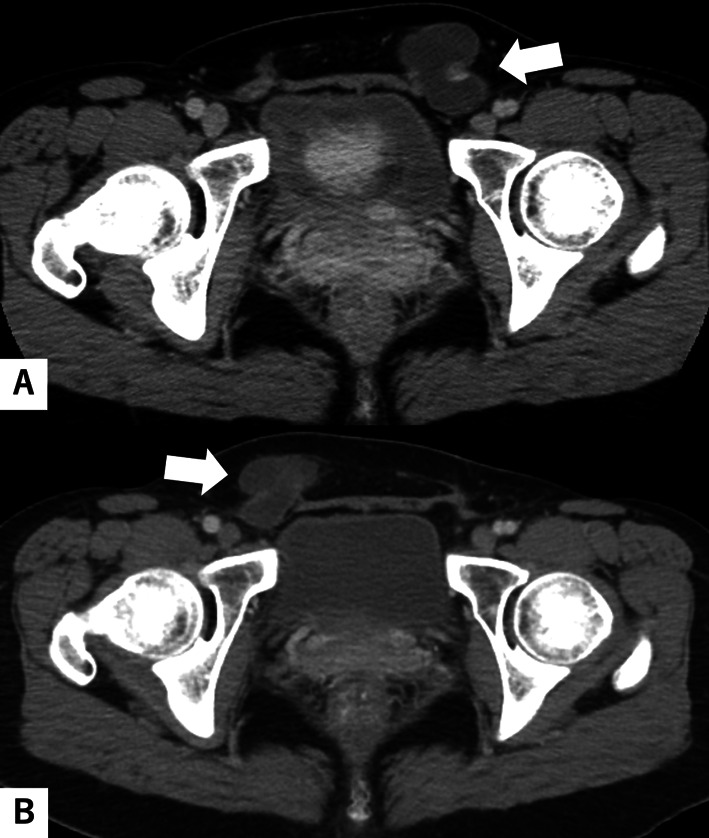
A Enhanced computed tomography (CT) images before the first surgery revealing multicystic lesions, suggesting a hydrocele of Nuck’s canal or inguinal hernia (arrow). No lesion was observed in the right inguinal area. B Enhanced CT images before the secondary surgery showing a multicystic lesion, suggesting a hydrocele of Nuck’s canal or a cystic tumor similar to that resected previously (arrow). No recurrence was noted in the left inguinal area
